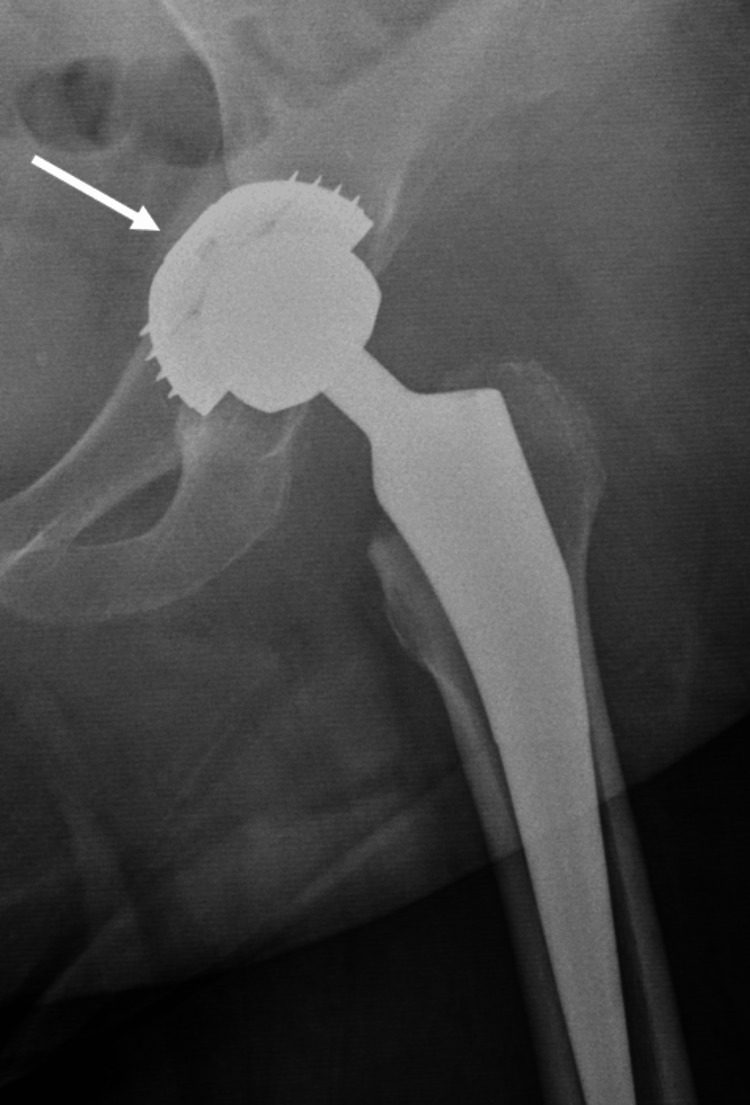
Anteroposterior X-ray view revealing an acetabulum roof fracture (white arrow) that occurred during the placement of the threaded cup
